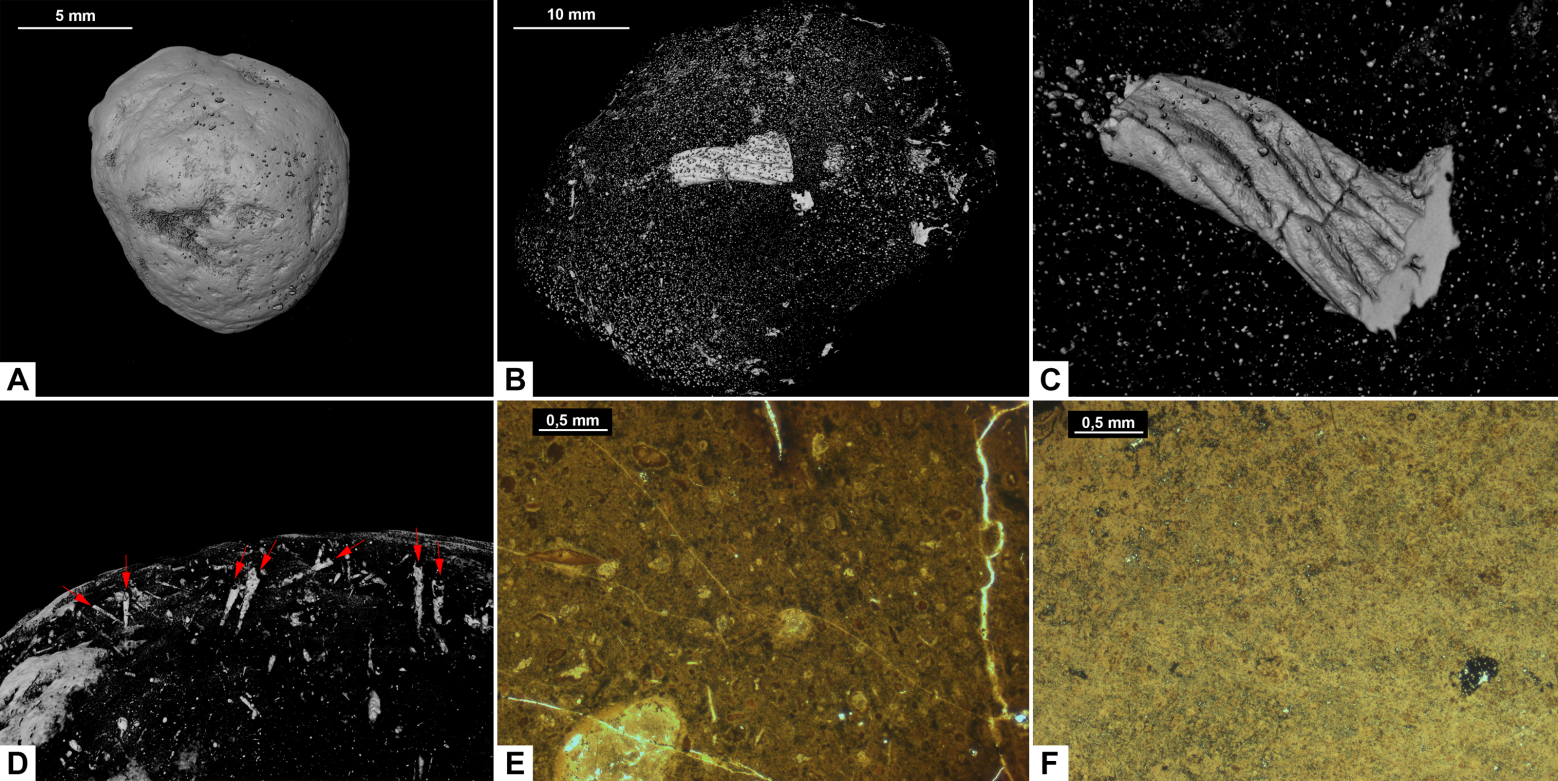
CT-scans (A–D) and thin sections (E–F) of morphotype C. (A) External view of morphotype C, PMO 250.271. (B) Internal view of PMO 250.277. (C) Unidentified fragment with length 10.7 mm, PMO 250.277. (D) Red arrows pointing to the entrance of cone shaped borings, PMO 250.0271. (E) Coarse matrix with degraded bone fragments, PMO 250.002. (F) Fine matrix with no inclusions, PMO 250.018.
